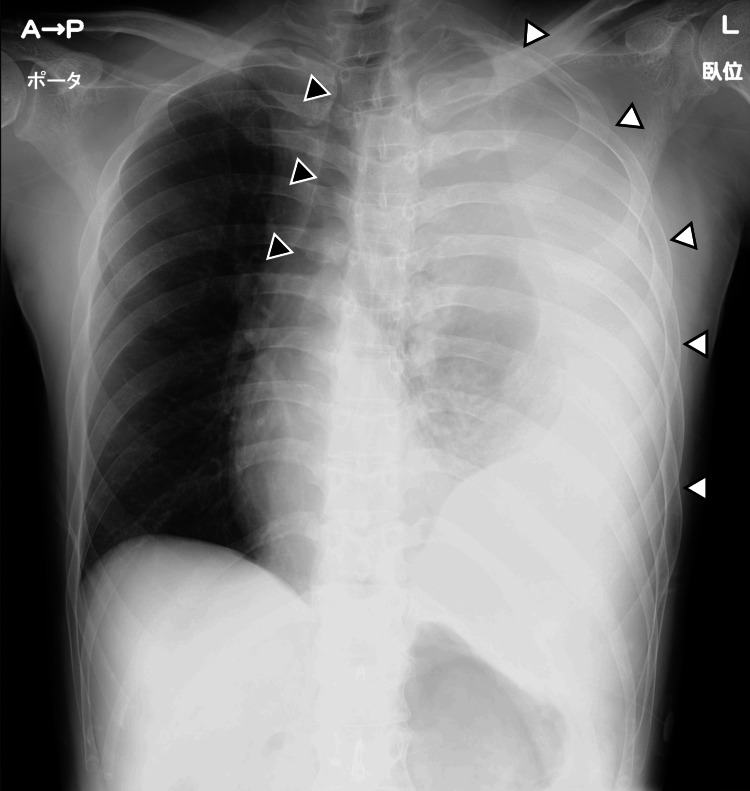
Chest X-ray Complete opacification of the left thorax (white arrows) and mediastinal shift toward the right (black arrows)
